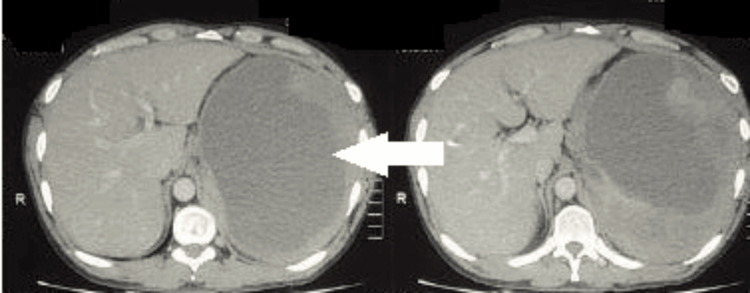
Intrasplenic pseudocyst rupture in chronic pancreatitis Axial CT abdomen showing a large hypodense cystic lesion within the upper pole of the spleen with signs of rupture (white arrow).
